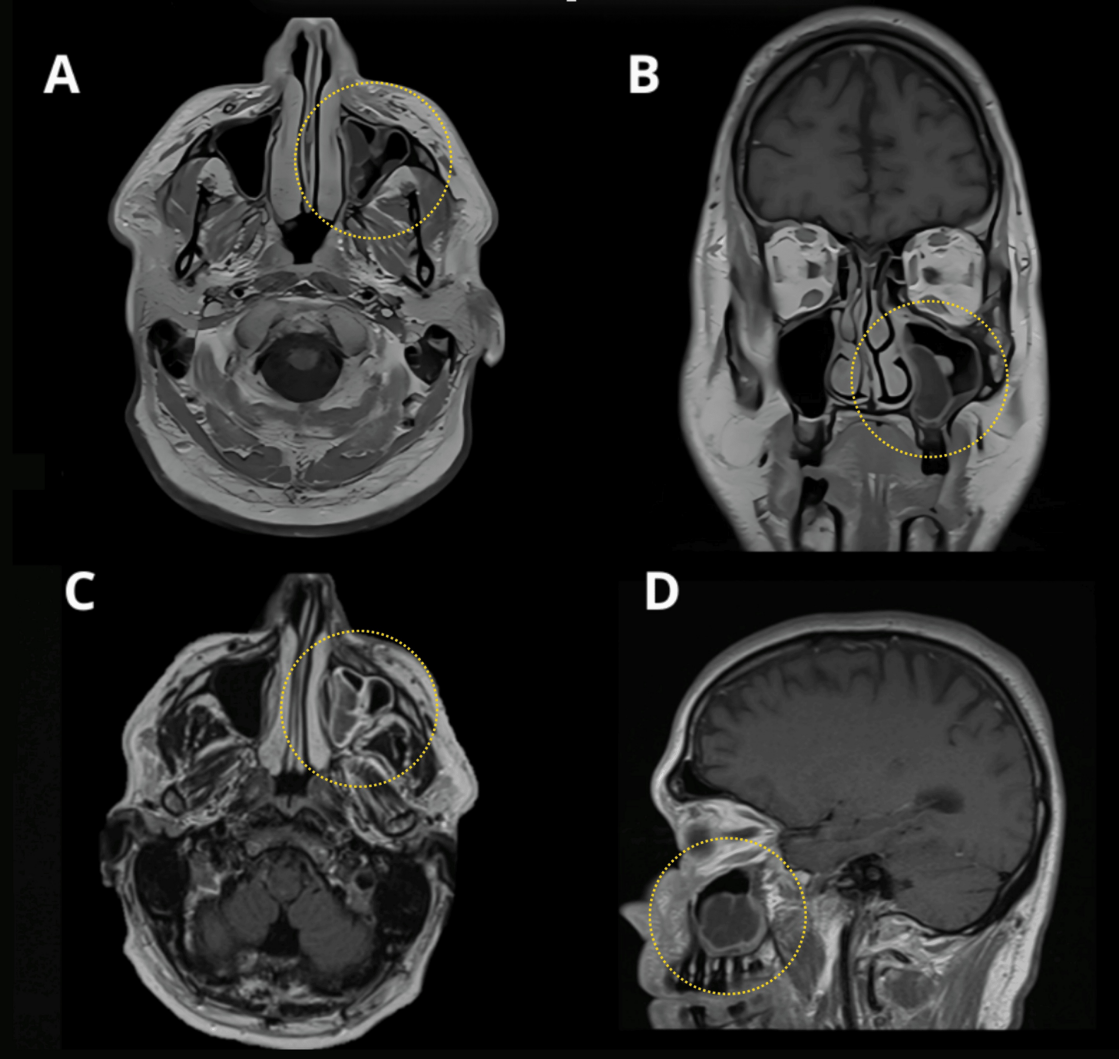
Pre-treatment head MRI with and without contrast. A. Axial T1-weighted image without contrast showing mucosal thickening of the left maxillary sinus.
